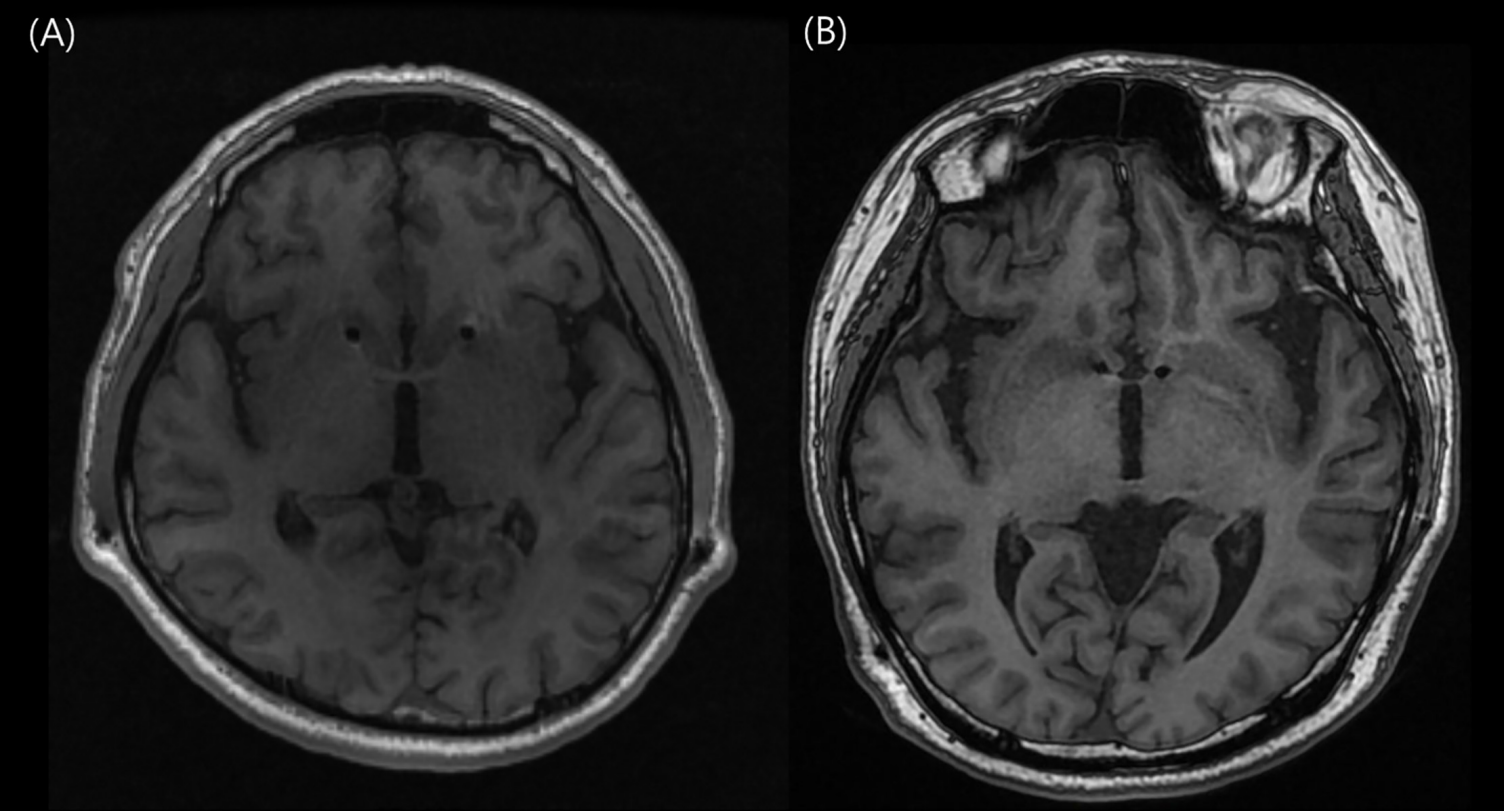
Postoperative magnetic resonance imaging after electrode insertion. (A) Anterior limb of internal capsule (B) Nucleus accumbens.
